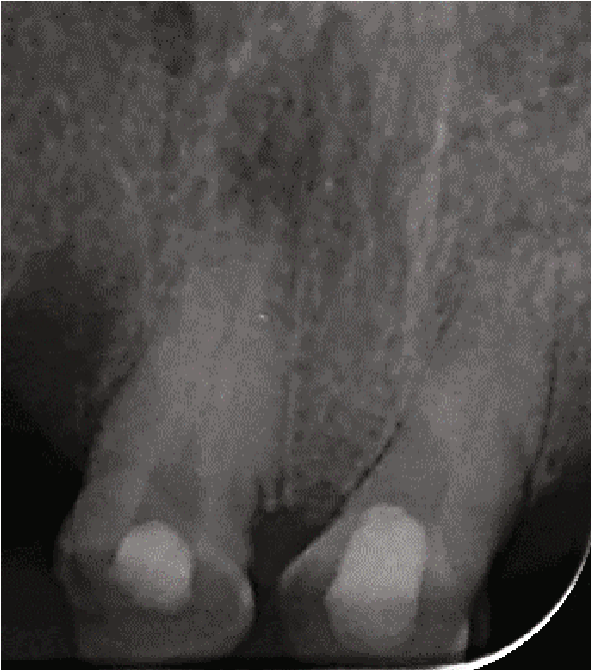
Initial radiograph of teeth #11 and #21.
